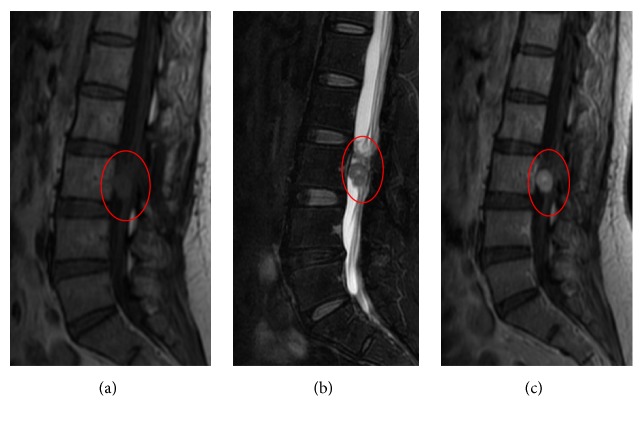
Images obtained from a 43-year-old woman with EMC of the spinal canal (red circle). MRI showed a mass in the spinal canal with both slight high signal on T_1_WI and T_2_WI (a, b). Gadolinium-enhanced T1-weighted fat-suppression images showed heterogeneous enhancement (c).
